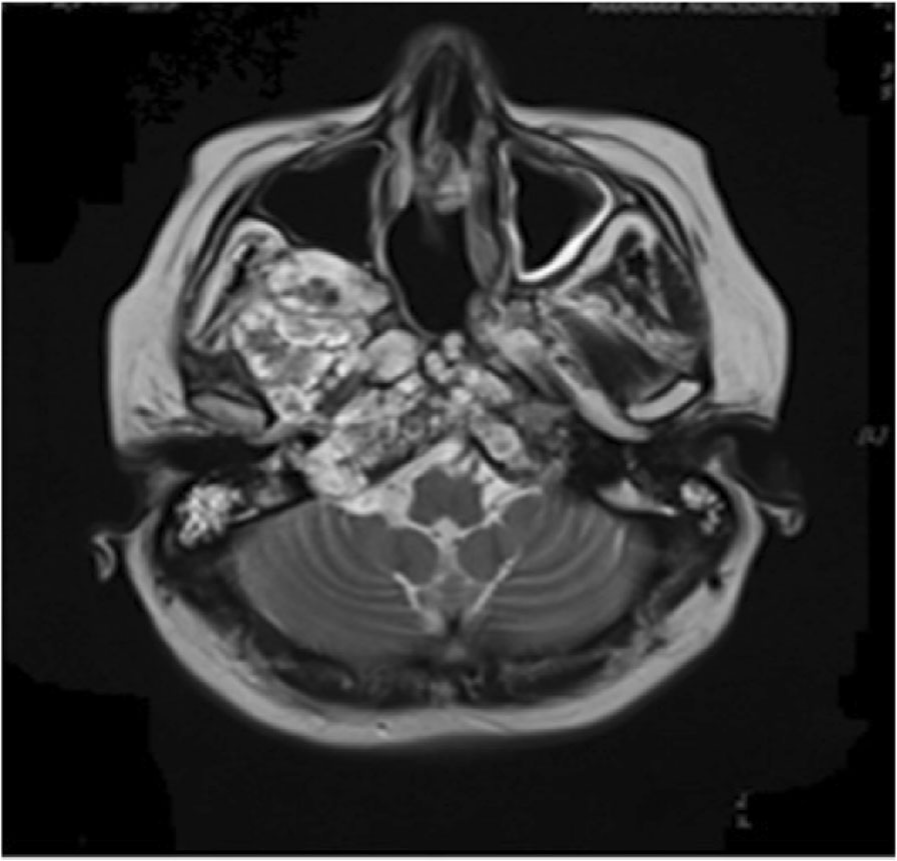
Magnetic resonance image of patient 3 treated with sunitinib (progressive disease 6 months)
